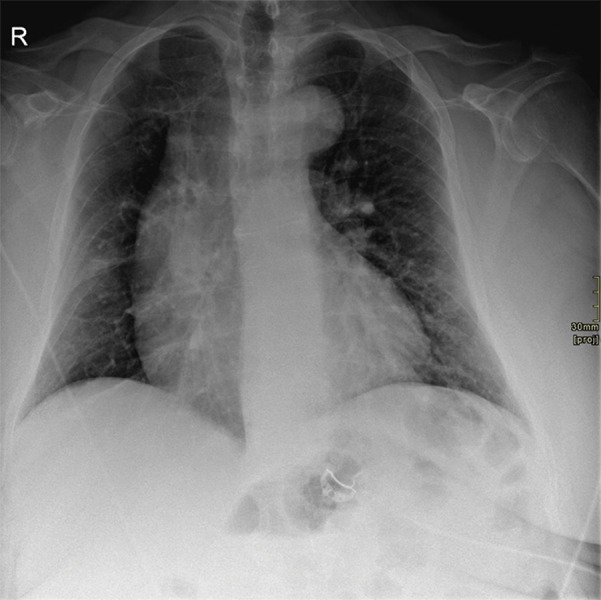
Chest radiography (frontal view) demonstrating cardiomegaly (cardiothoracic ratio = 0.59). Pulmonary vascular markings are globally increased, without evidence of consolidation.
